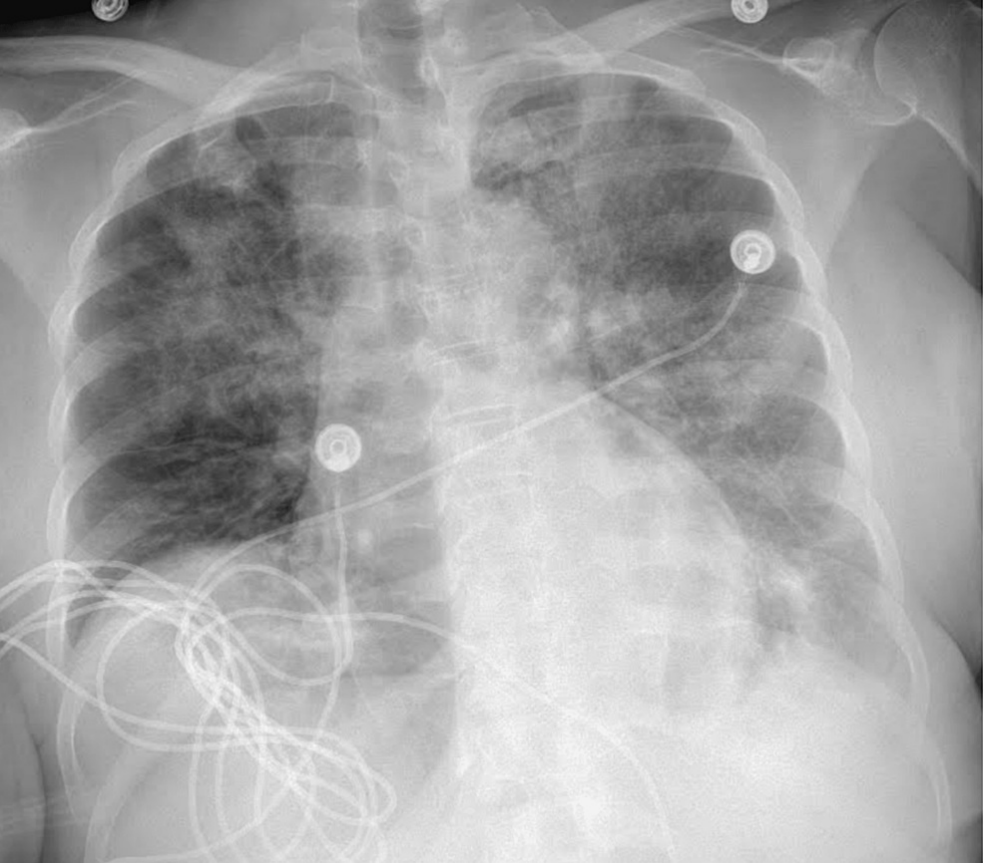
X-ray taken one day after starting a steroid regimen. Although bilateral parenchymal opacities are still present, there is a significant improvement compared to Figure 1.
